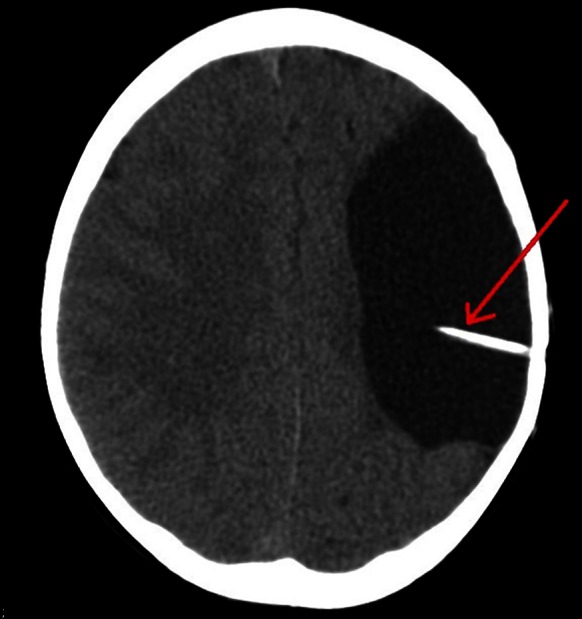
CT scan showing the shunt inside of the arachnoid cyst
